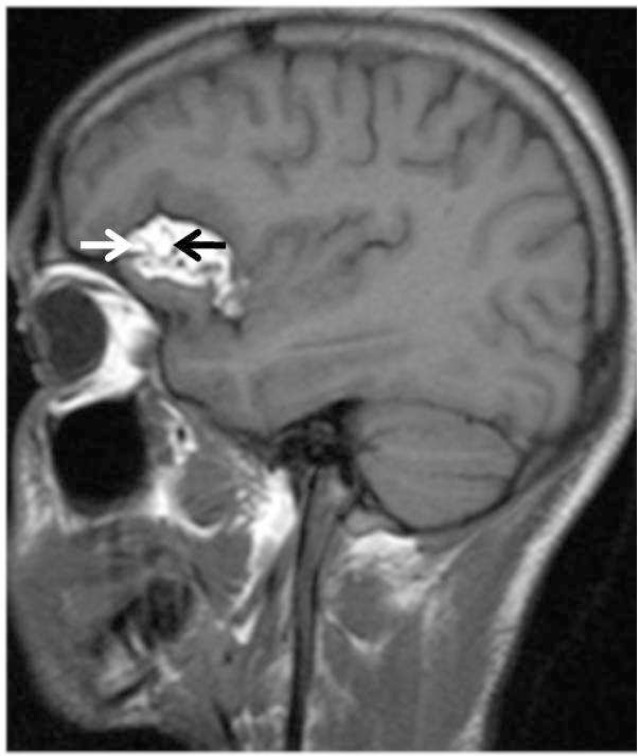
T1-weighted right parasagittal image shows the lesion having typical T1 shortening with bright signal specific of lipomas (white arrow). Multiple linear flow voids are also seen within the lesion (black arrow).
